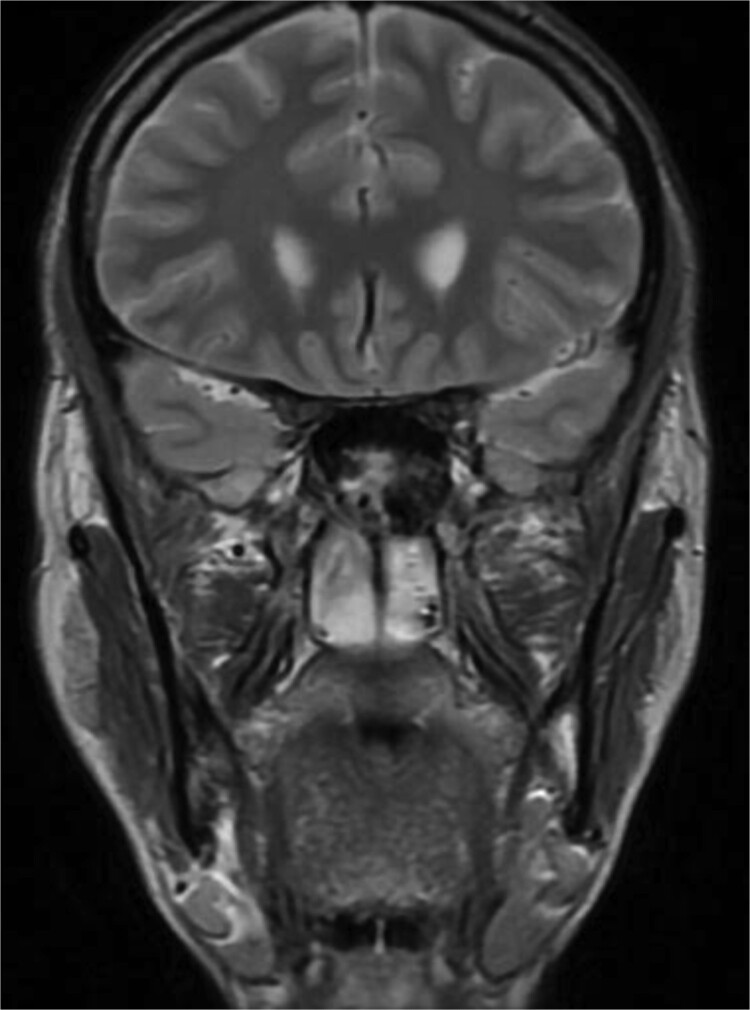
MRI of paranasal sinuses shows the sphenoid sinus is expanded and bulging superiorly into the pituitary fossa causing displacement of pituitary gland and stalk without mass effect upon the optic chiasm. It extends laterally causing effacement of the cavernous sinus.
